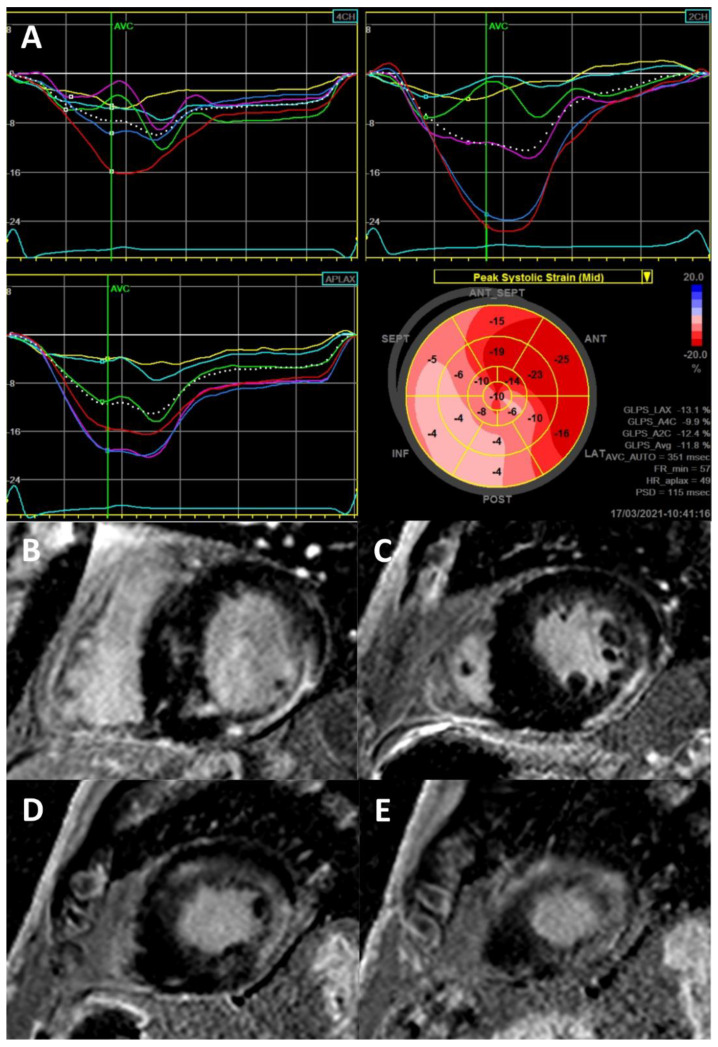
Strain echocardiography for the detection of LGE. (A) Transthoracic echocardiogram with 2D-strain analysis by speckle tracking showing reduction of longitudinal strain, particularly in the inferolateral, inferior and inferoseptal walls and apex, in a 73-year-old male Fabry patient; (B–E) Cardiac MRI (3.0 Tesla) with LGE in short-axis slices, from the LV base to the apex, showing fibrosis in the same regions where the reduction in longitudinal strain was more pronounced.
